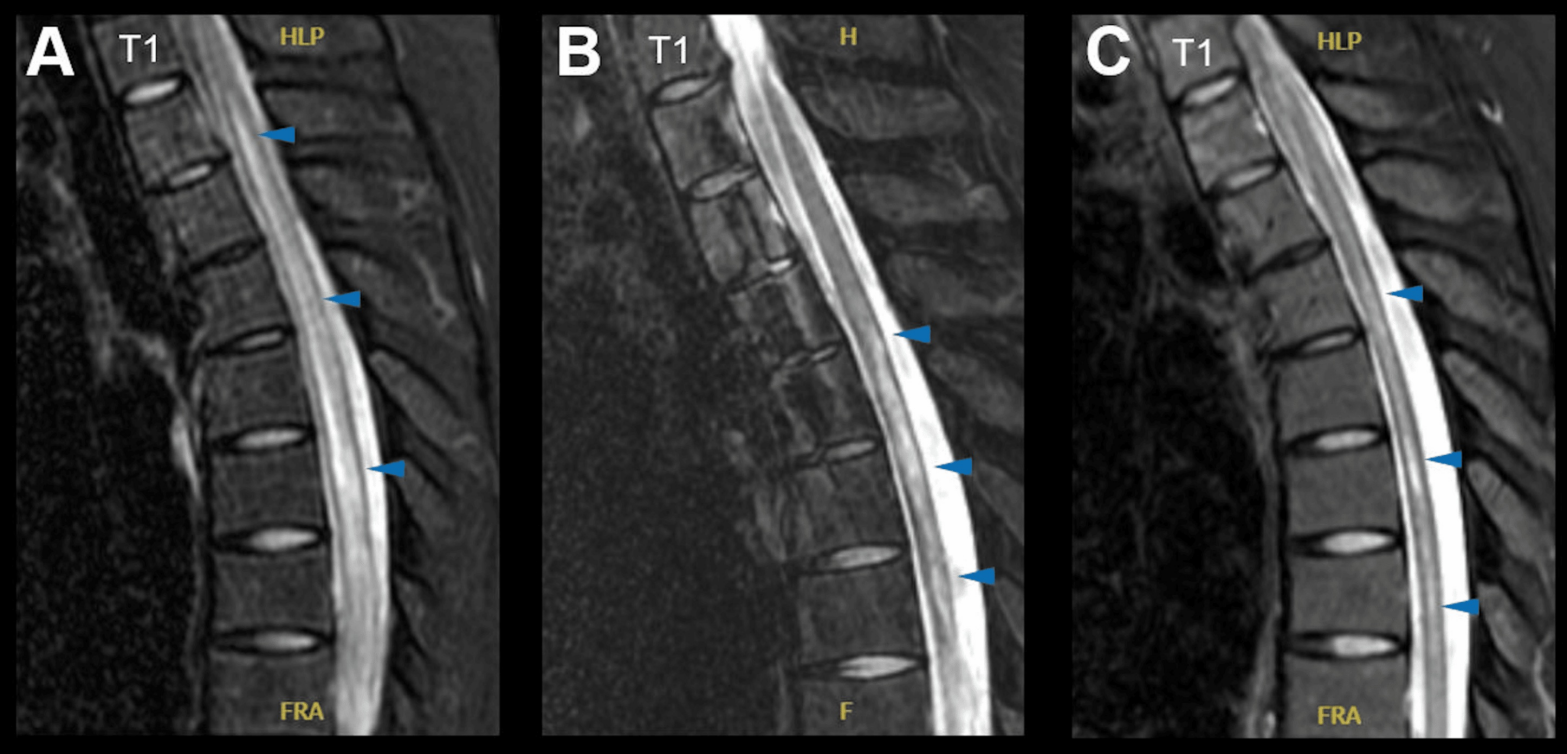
Magnetic resonance imaging findings. Longitudinally extensive thoracic transverse myelitis on T2-weighted sagittal magnetic resonance imaging at admission (A). The spinal cord lesions shrank, and swelling reduced after one month (B). The spinal cord lesions further reduced in size after nine months (C).
